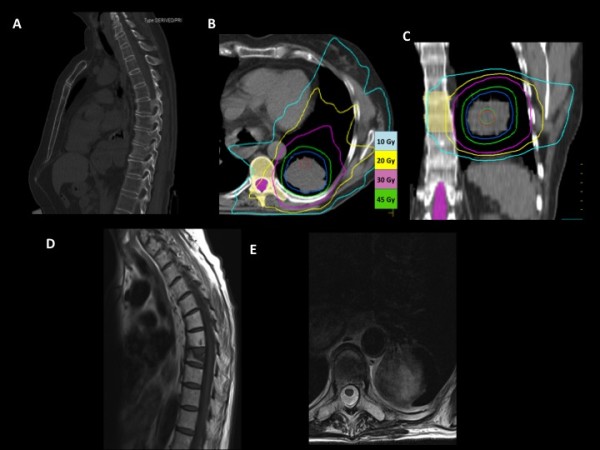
Sagital view of CT slide before SBRT (A). Transverse (B) and coronal (C) view of SBRT dose distribution in the lung metastases. Blue, yellow, and pink lines represent the 10 Gy, 20 Gy and 30 Gy isodose lines, respectively. The 10 Gy isodose distribution shapped the 7^th^-8^th ^vertebral fractures. (D) Sagittal and coronal (E) view of MRI ten moths before SBRT.
